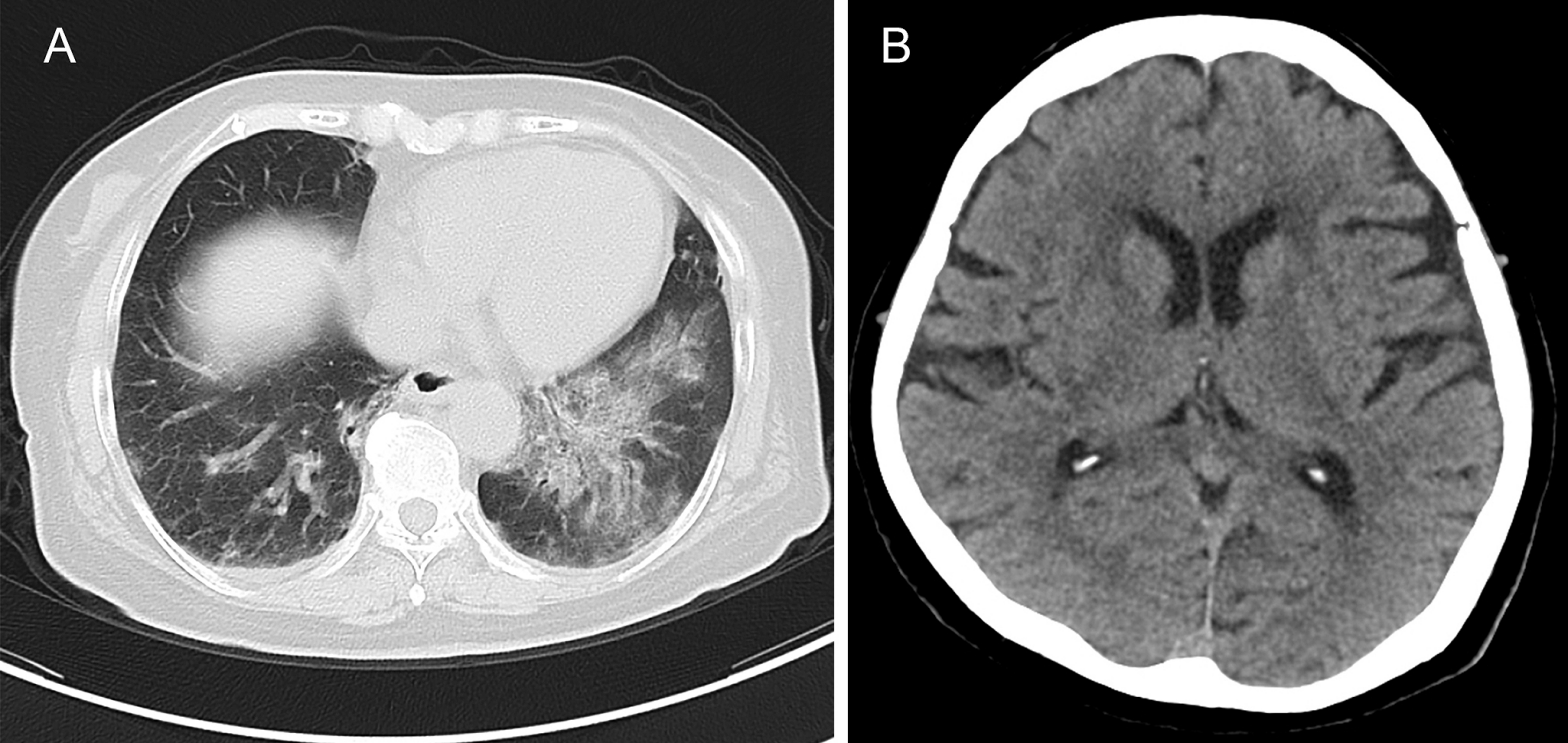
Computed tomography images of the chest and head. A) Chest computed tomography (CT) image taken on admission shows slightly left and bottom dominant ground-glass appearance and pleural fluid. B) Head CT images taken on the same day show no obvious infarction or hemorrhage.
